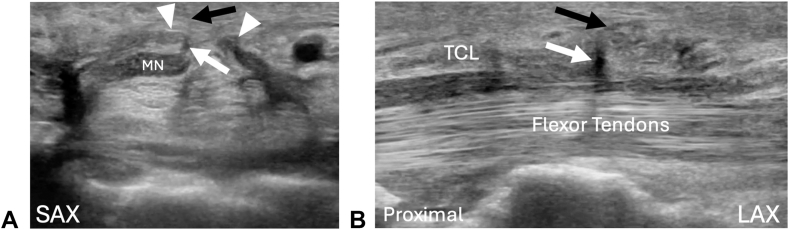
Six week follow-up ultrasound. Short-axis or anatomic transverse and LAX ultrasound or anatomic sagittal images showing a released TCL complex and an intact TLB of the MN. A SAX image at the midcarpal tunnel (comparable to Figs. 1A and 2) showing transection of the TCL complex (white arrowheads). Note the palmar or superficial position of the MN relative to Figure 1A. Once again, the TLB is seen branching from the ulnar side of the MN (white arrow) and traversing into the subcutaneous tissue (black arrow). B LAX view of the carpal tunnel (comparable to Fig. 1B) showing an intact TLB branching from the MN in the midcarpal tunnel (white arrow) and traversing through the TCL complex and into the subcutaneous tissue (black arrow).
